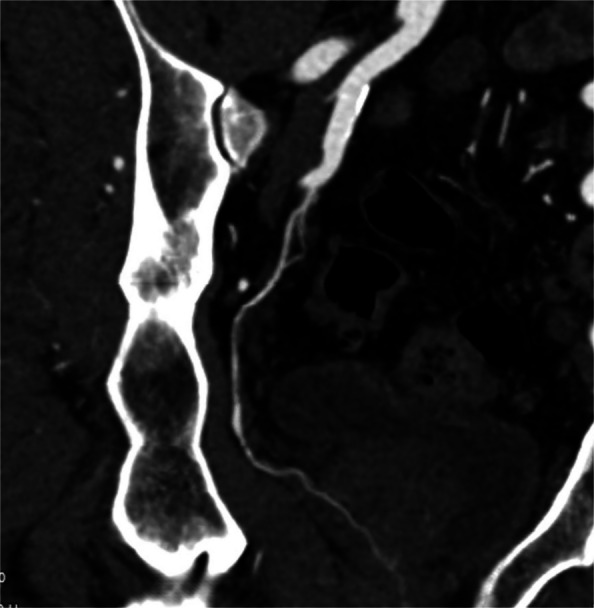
Prostate artery identification of planning CTA
